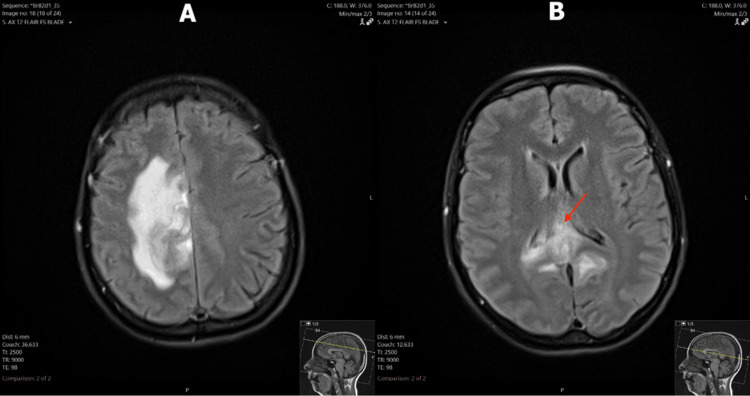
T2-weighted FLAIR axial MRI images of the brain (A) Irregular, heterogeneous FLAIR signal in the right cingulate gyrus/frontoparietal region with adjacent FLAIR hyperintensity representing vasogenic edema. (B) Poorly defined, heterogeneous, mildly hyperintense FLAIR signal in the splenium of the corpus callosum with adjacent subependymal involvement (arrow). FLAIR: fluid-attenuated inversion recovery; MRI: magnetic resonance imaging
